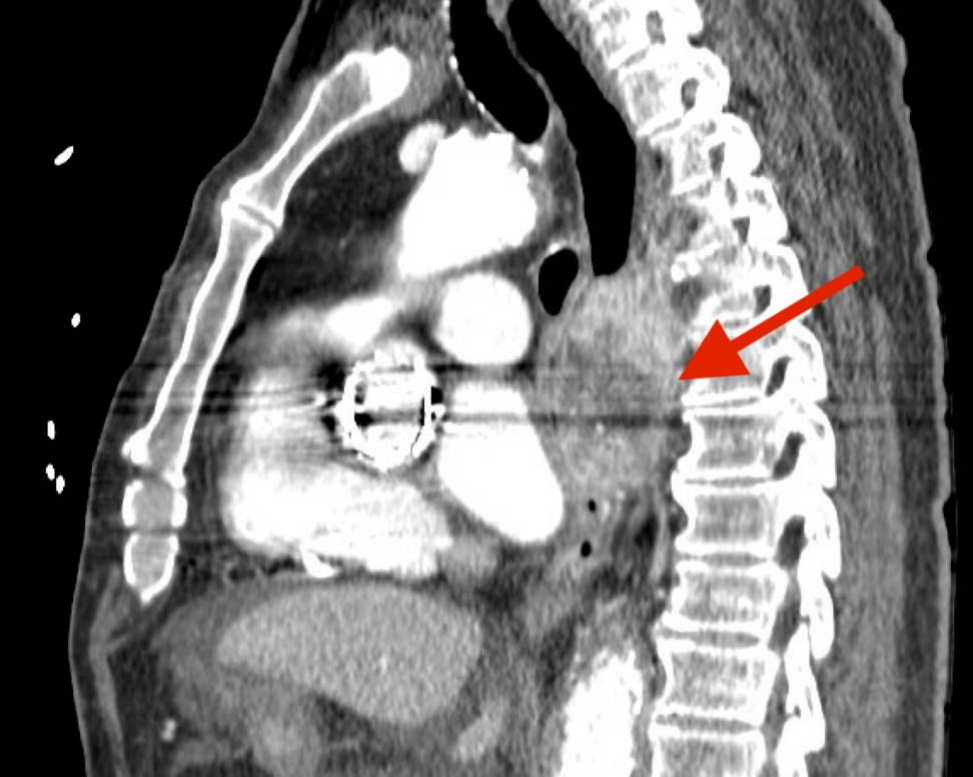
Sagittal view of the chest by contrast-enhanced computed tomography scan. The figure highlights an esophageal mass causing significant luminal obstruction and compression of adjacent structures (red arrow).
